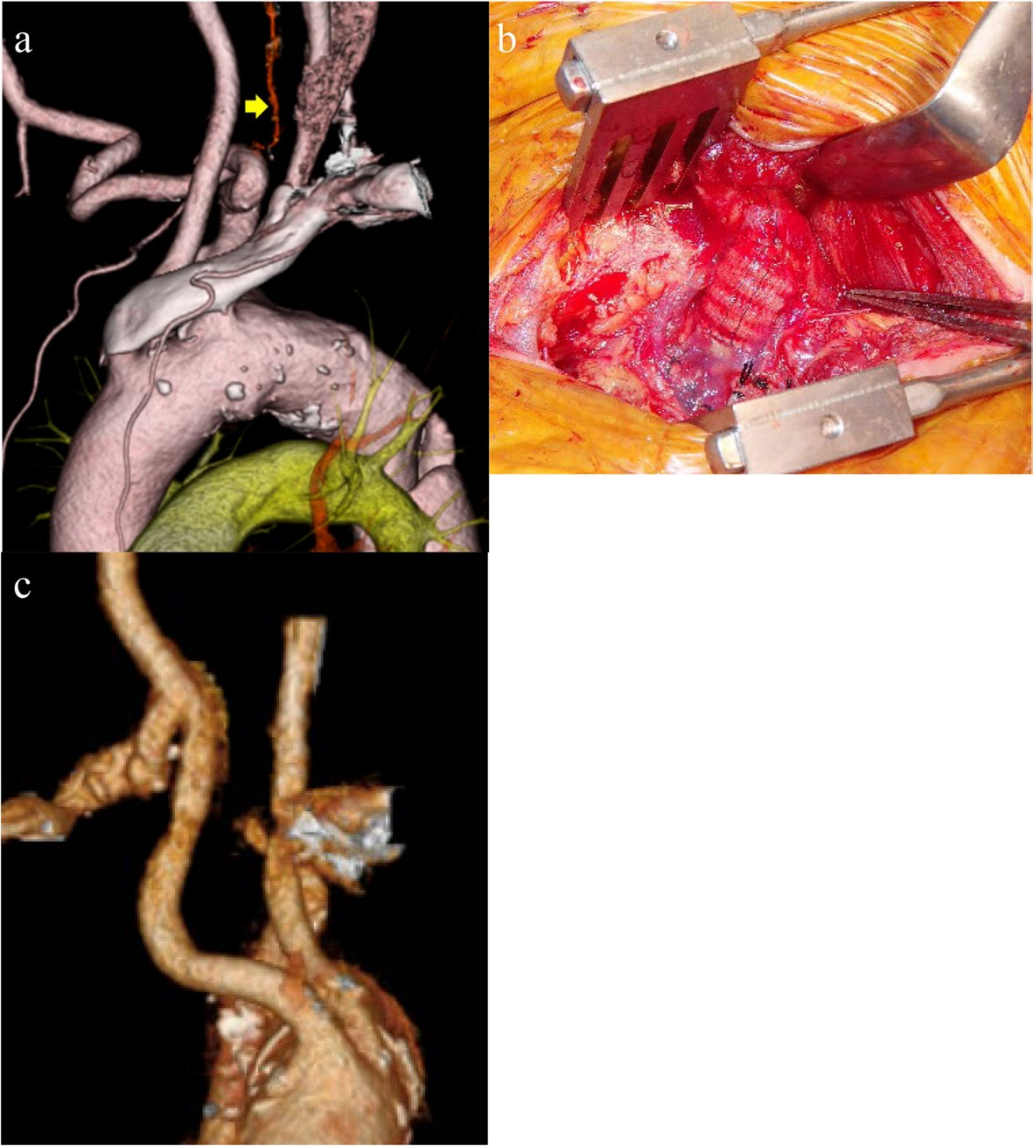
a 3D computed tomography reconstruction shows the right vertebral artery branched from the aberrant right subclavian artery (yellow arrow). b Intraoperative findings: right carotid artery-to-aberrant right subclavian artery bypass using an 8-mm Dacron graft (black arrow). c Postoperative computed tomography reveals a patent bypass graft (White arrow)
